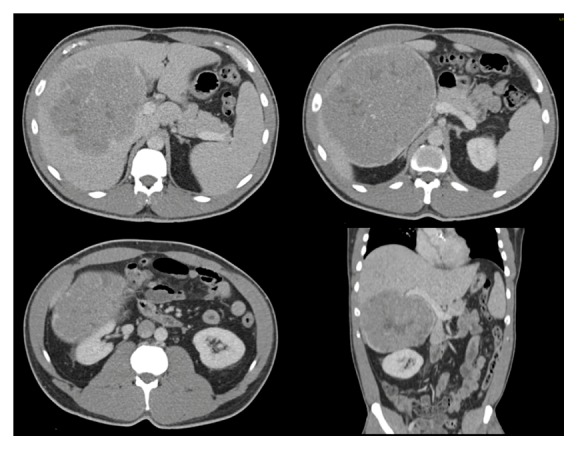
Abdomen and pelvis CT that shows a 16 cm mass in the right lobe of the liver, with mass effect on the surrounding tissues, with compression of the IVC.
